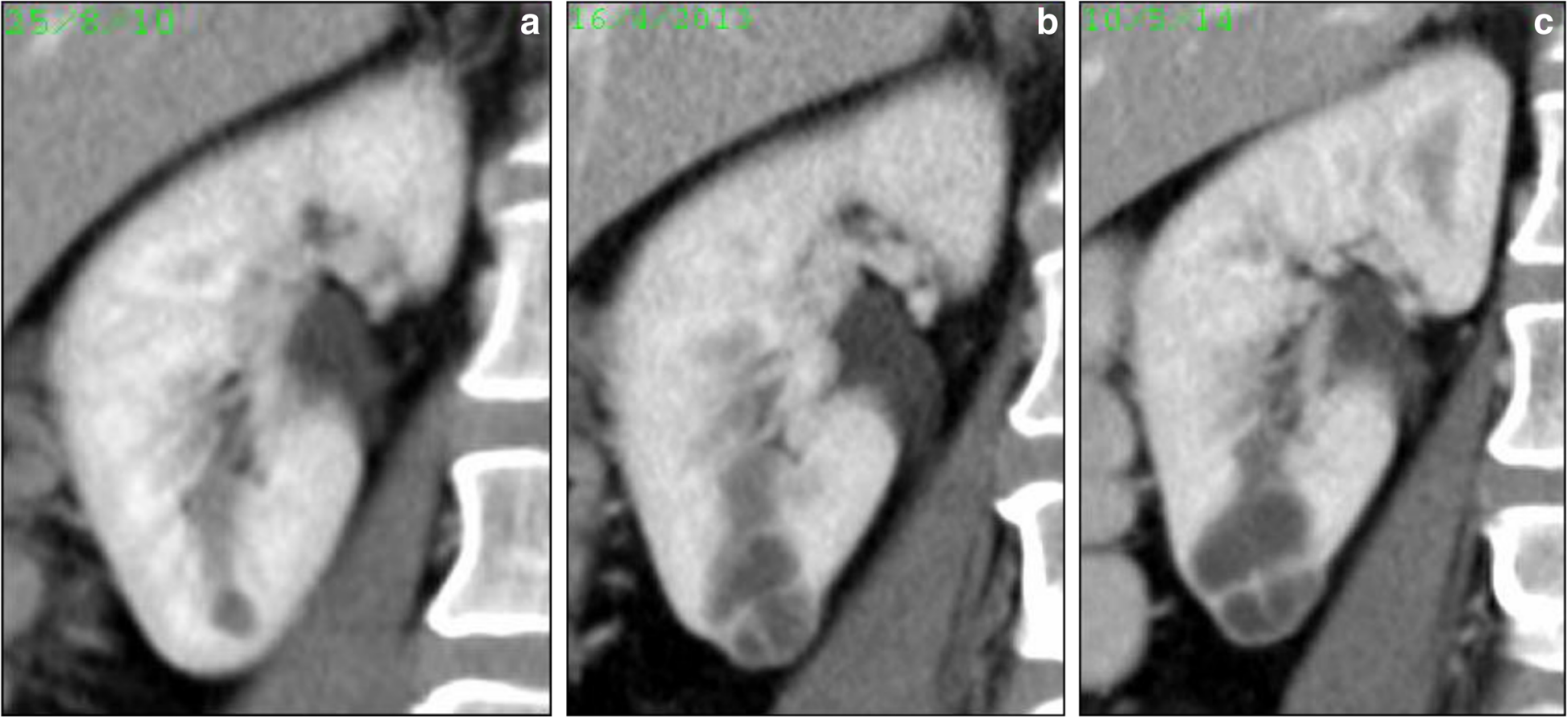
Ongoing enlargement of CARC: Coronal CT images show continued slow enlargement of a right lower pole renal cyst, 6 mm at baseline in July 2010 in patient 10 on crizotinib over 45 months from start of treatment at time points August 2010 (a), April 2013 (b) and May 2014 (c)
